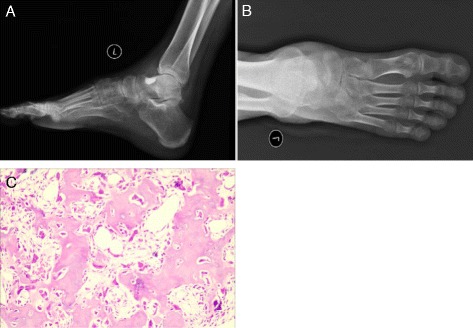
Postoperative imaging of the left ankle after the second surgery. (a, b) Postoperative radiographs of the left ankle show that the nidus had been completely removed. (c) Postoperative pathologic examination confirmed that the lesion was an osteoid osteoma (100×)
